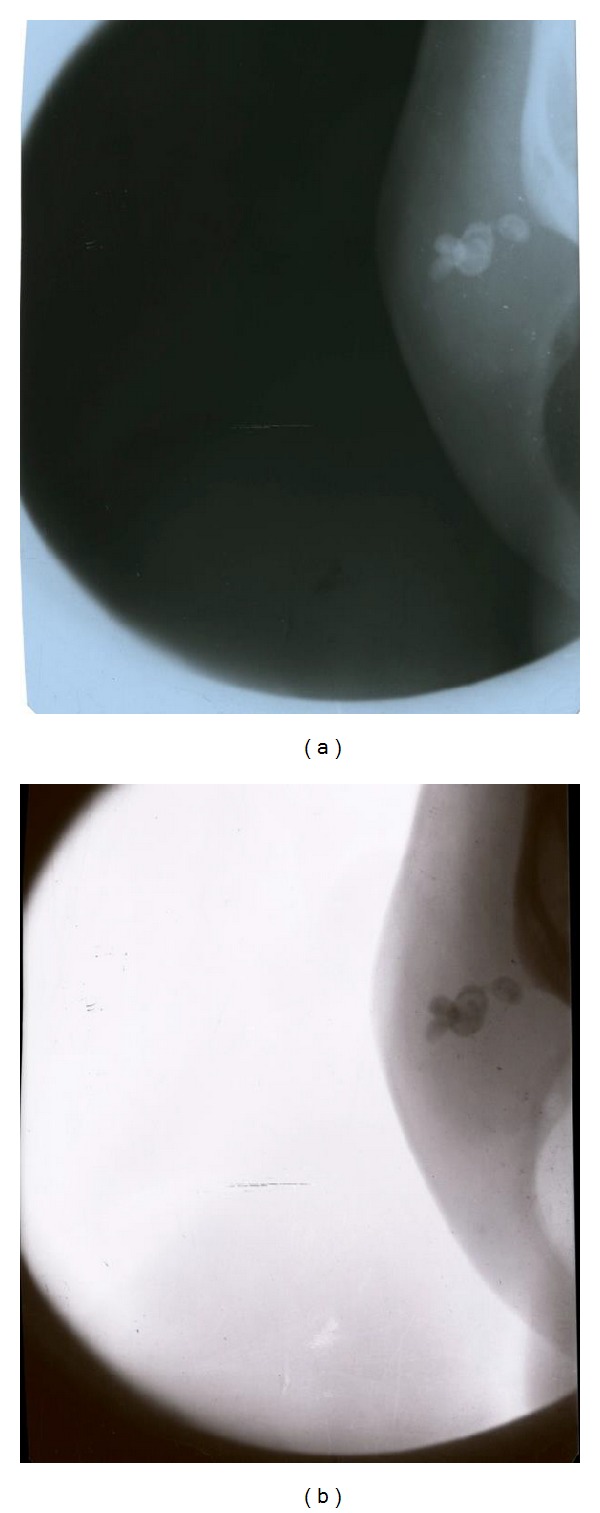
Puffed cheek radiograph and its inverted image depicting the calcifications noted within the lesion.
